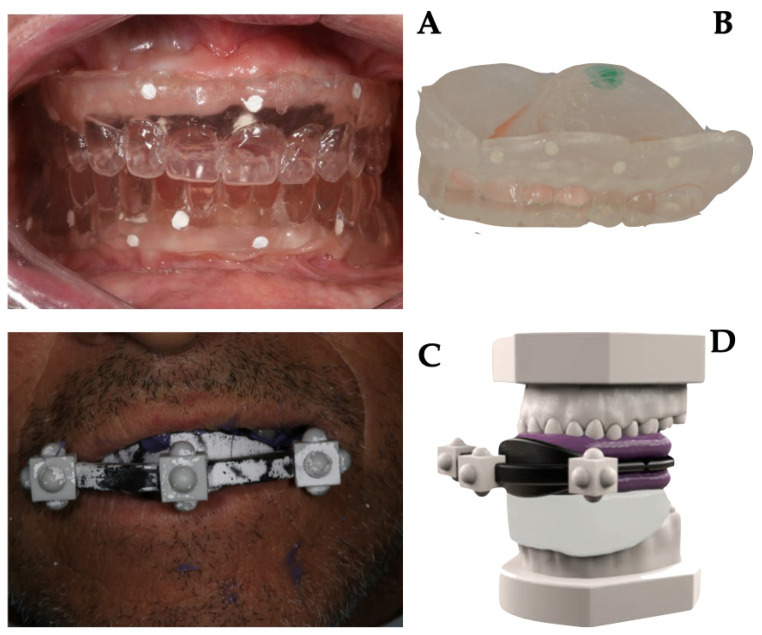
Data acquisition protocol: (A) intraoral position of resin duplicates of patient’s prosthesis. Gutta-percha markers were inserted into 1 mm deep niches on the prosthesis (on the vestibular and palatal–lingual sides). The patient underwent CBCT (Cone Beam Computed Tomography) scanning while wearing the denture; (B) a second CBCT of the denture alone was performed; (C) a duplicate of the prosthesis was attached to an extraoral stent with three-dimensional radiopaque marks. The whole was stabilized in the mouth with a radiolucent occlusal index. The patient underwent CBCT scanning while wearing the duplicate, stent, and stabilization index; (D) extraoral scans were performed using a laboratory scanner of all collected data.
